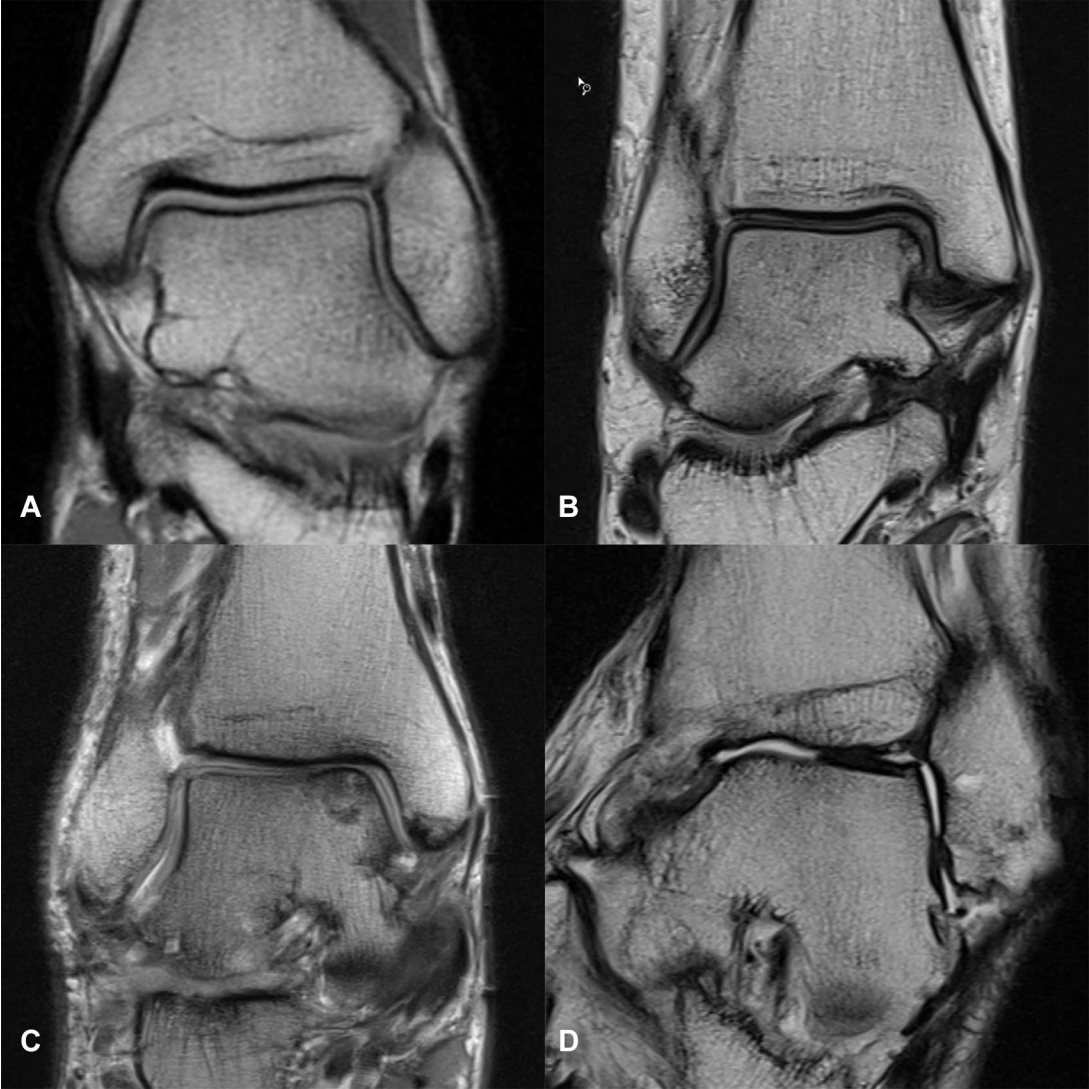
Grading of deep cartilage damage on proton density–weighted magnetic resonance images of 4 different patients: (A) none, (B) mild, (C) moderate, and (D) severe.
